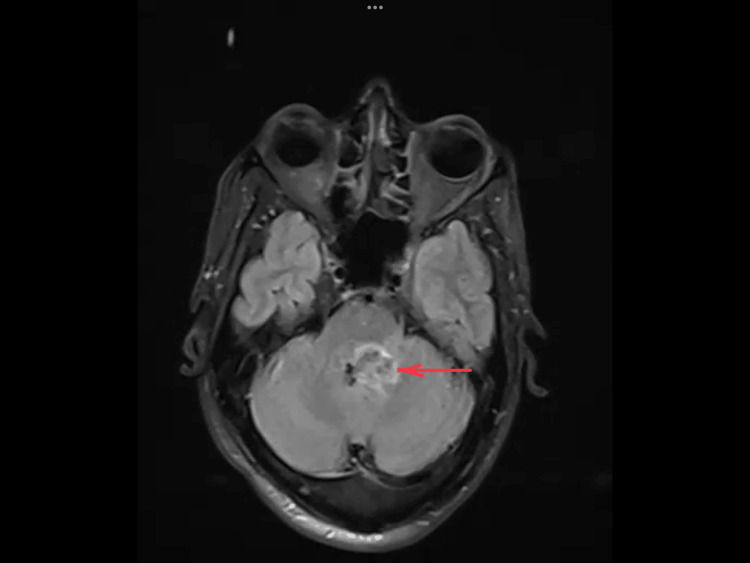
MRI brain: T2 FLAIR image showing hyperintense area involving the left posterior aspect of the hemipons extending to the left middle cerebellar peduncle and into the adjacent cerebellum, indicating hemorrhage of different stages pointed using red arrows FLAIR: Fluid-attenuated inversion recovery; MRI: magnetic resonance imaging
